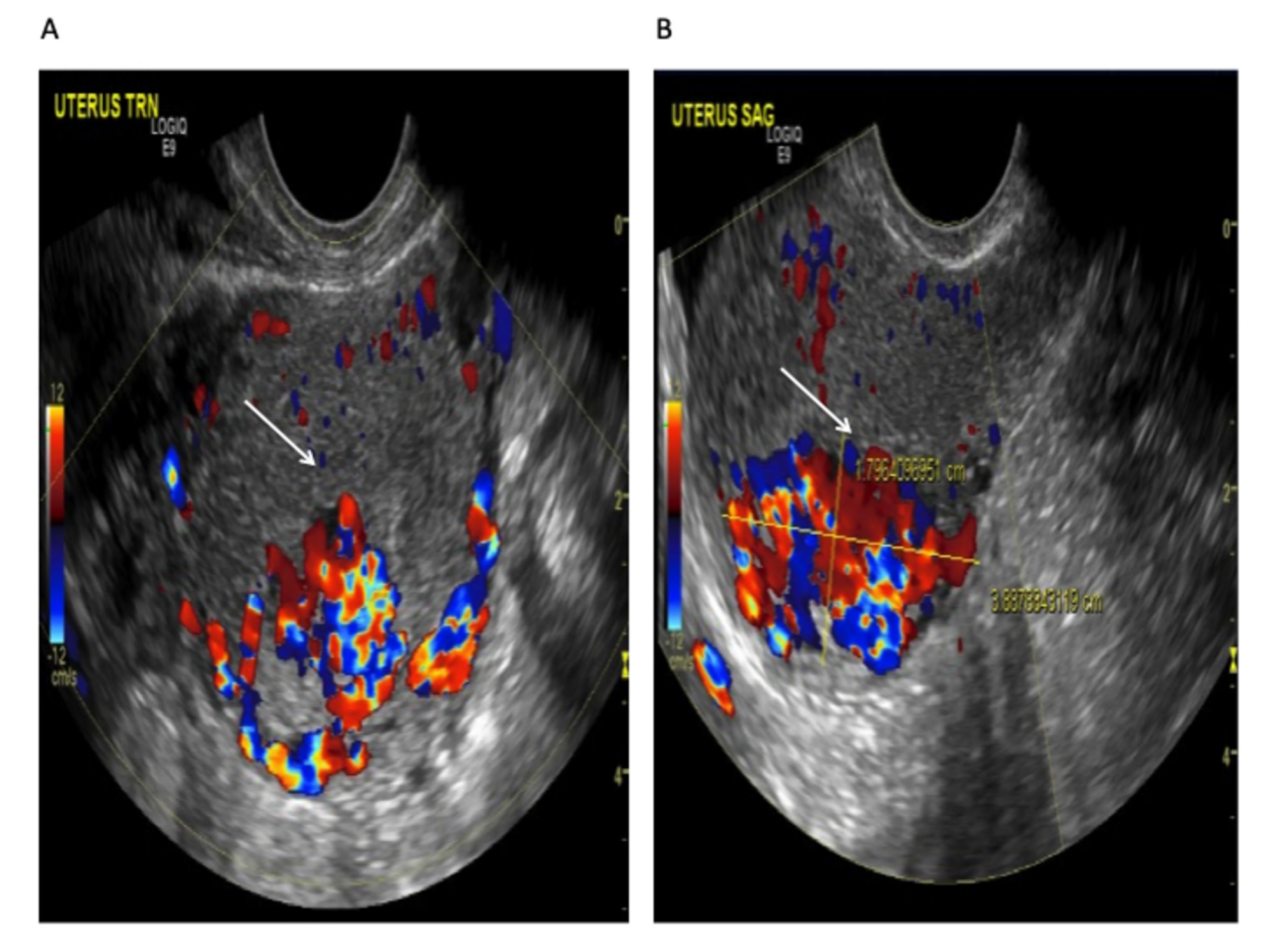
Transvaginal ultrasound of the uterus with Doppler revealing a hypervascular lesion: (A) transverse view (B) sagittal view
